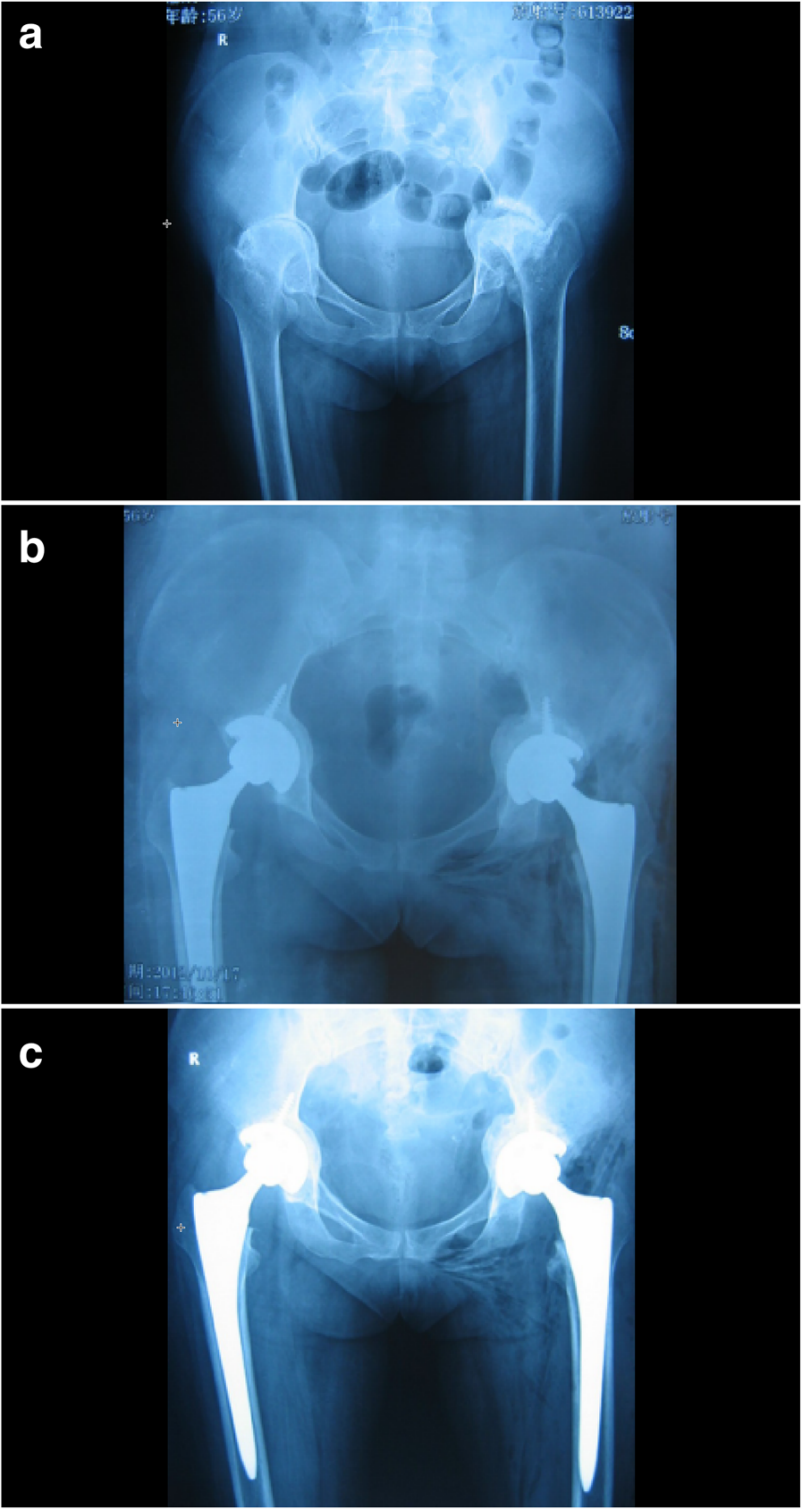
a A bilateral acetabular protrusion developing secondary to RA; the bilateral femoral head protruded inward over Nelaton’s line. b Single-stage not session session bilateral total hip arthroplasty (with the placement of a titanium-coated, biopsy acetabular cup; Smith & Nephew, USA) accompanied by acetabular reconstruction using autologous bone. An X-ray taken immediately after surgery showed that the rotational center of the hip joint had returned to the normal anatomical location. The initial stability of the acetabular cup was good. c At the 4-year follow-up, the X-ray revealed complete healing of the bilateral bone graft, without bone resorption, an acetabular protrusion, or loosening
